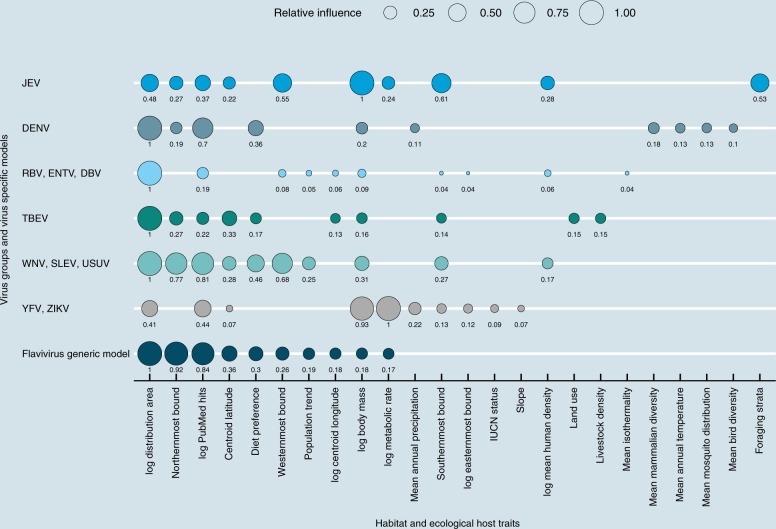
Macro-ecological traits of flavivirus hosts. Plot describing the ten most important biological and ecological host traits and their relative influence for all models predicting flavivirus hosts. YFV, yellow fever virus; ZIKV, Zika virus; WNV, West Nile virus; SLEV, St. Louis encephalitis virus; USUV, Usutu virus; TBEV, Tick-borne encephalitis virus; RBV, Rio Bravo virus; ENTV, Entebbe bat virus; DBV, Dakar bat virus; DENV, dengue virus; JEV, Japanese encephalitis virus
